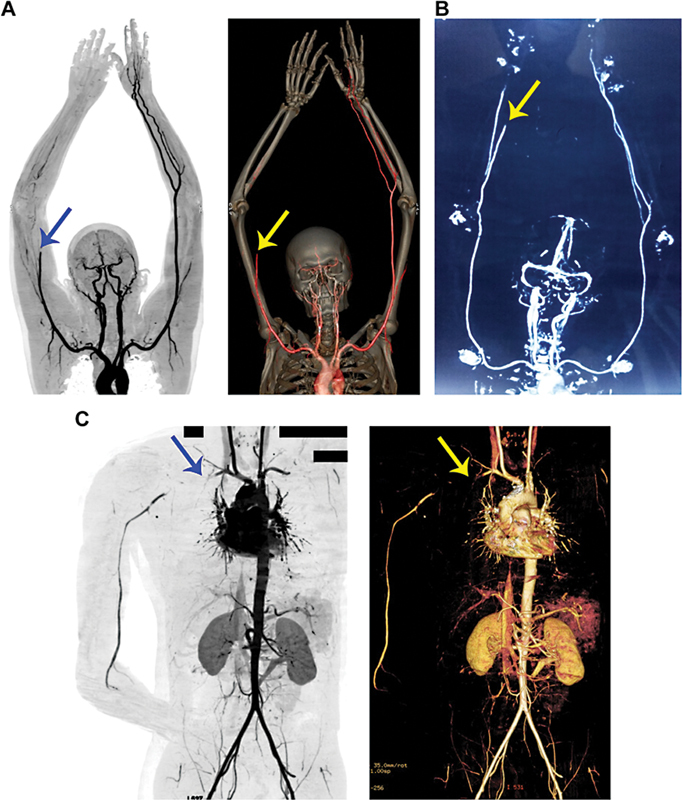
CT angiography reveals occluded peripheral arteries and a lack of downstream blood flow in Russell's bite victims. (
